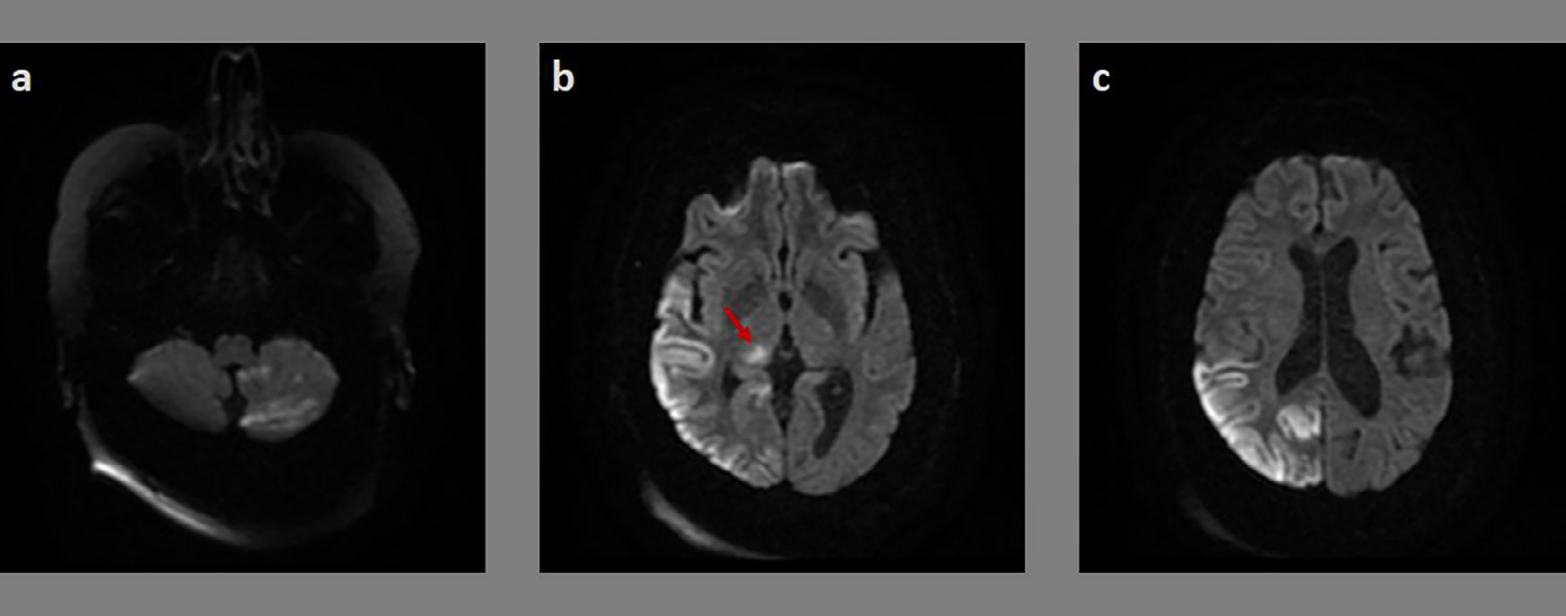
Axial brain DWI MRI showing increased DWI signal in the left cerebellar hemisphere (A), the right temporal lobe and pulvinar thalamic nuclei (red arrow) (B), and the right occipital lobe (C). (Color version of figure is available online.)
